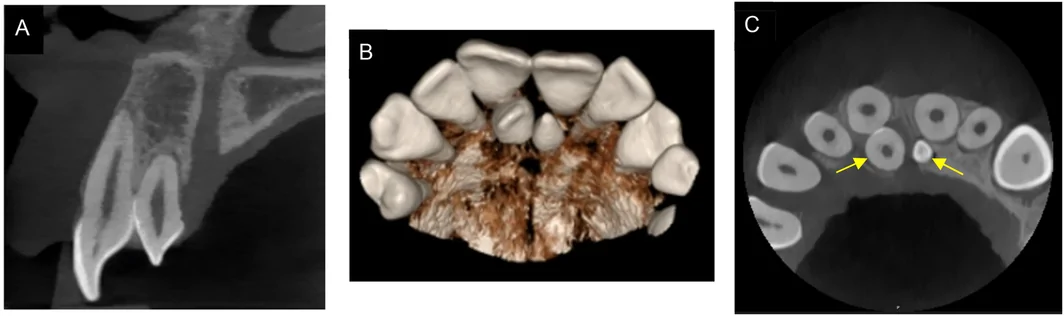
CBCT images showing (A) A sagittal view of the fusion. (B) A 3D view of the fusion and anterior maxillary dentition from the palatal aspect. (C) An axial view of the fusion and anterior maxillary dentition.
